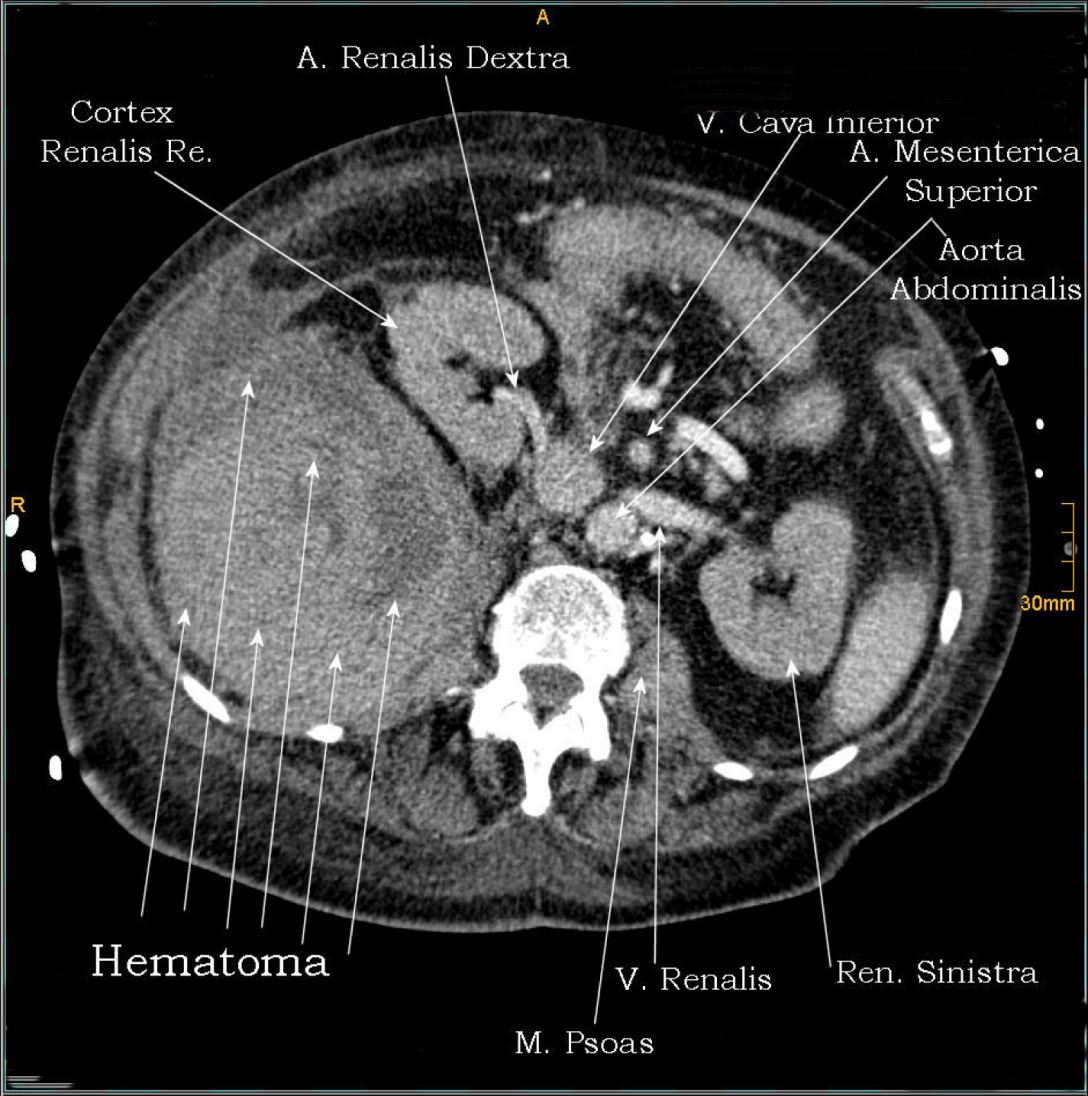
3^rd ^case. CT - axial plan of a huge hematoma shifting the whole right renal to the middle line.
